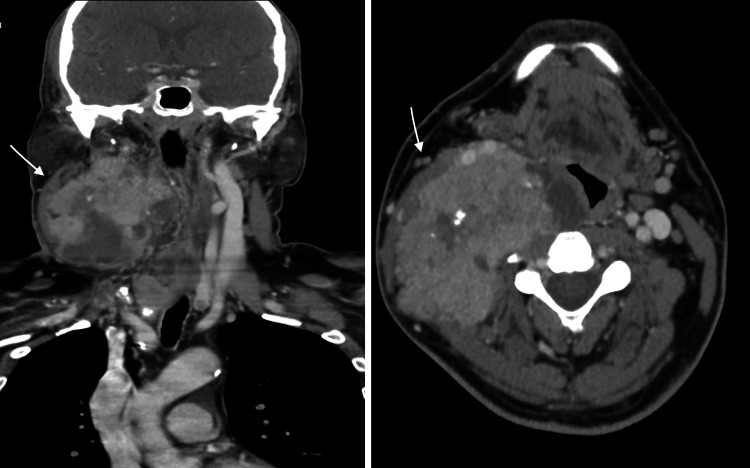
Neck CT axial and coronal views at initial evaluation The images show both thyroid lesions and the lymph node mass with significant displacement of adjacent structures, including the larynx, hypopharynx, and right carotid sheath CT: computed tomography
